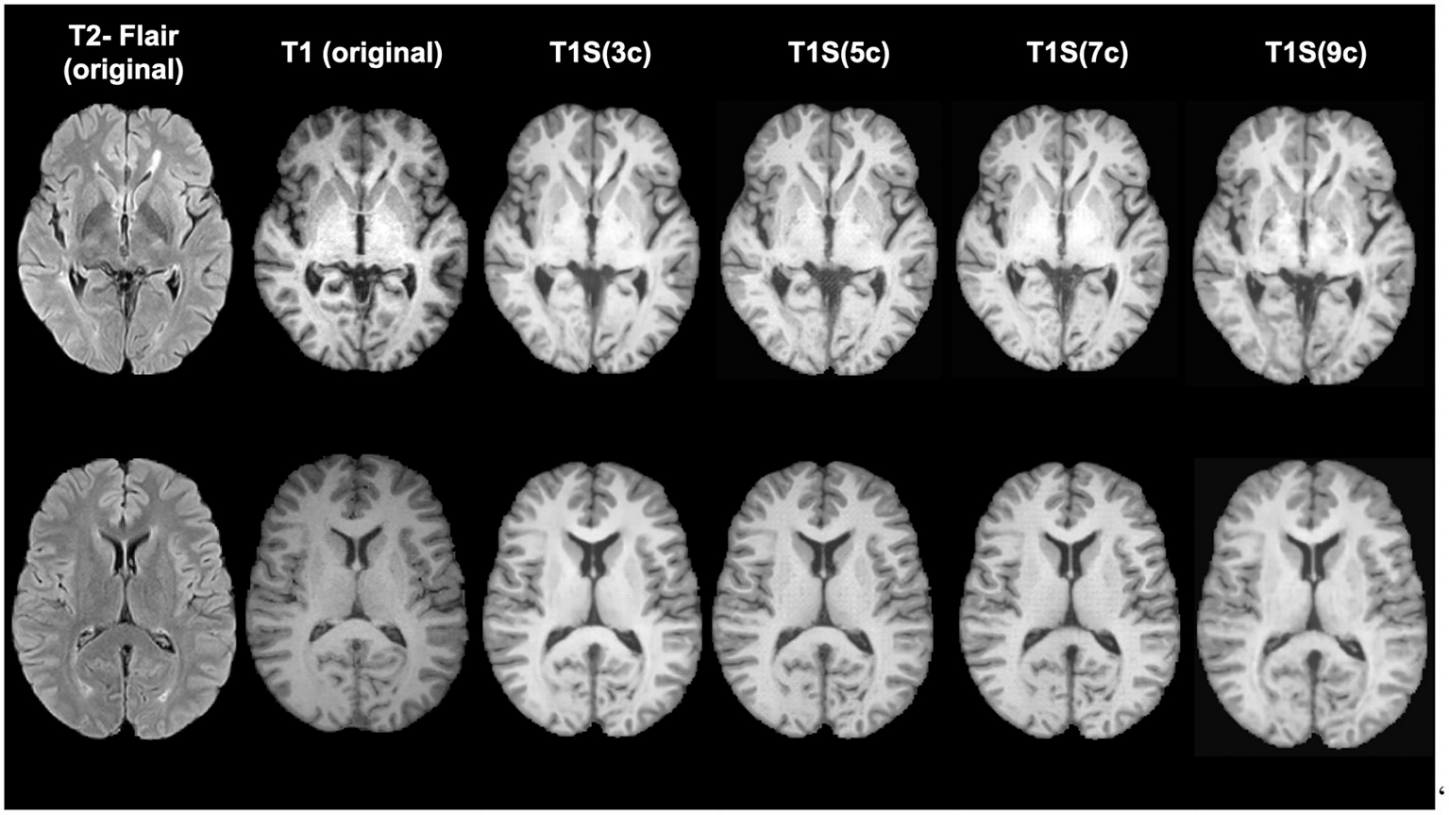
Examples of original and synthetically obtained images. The first column shows the original T2-FLAIR image, while the second column shows the original T1-w image. The following columns show the T1-w images obtained from the T2-FLAIR image using a different number of clusters (3, 5, 7, and 9).
